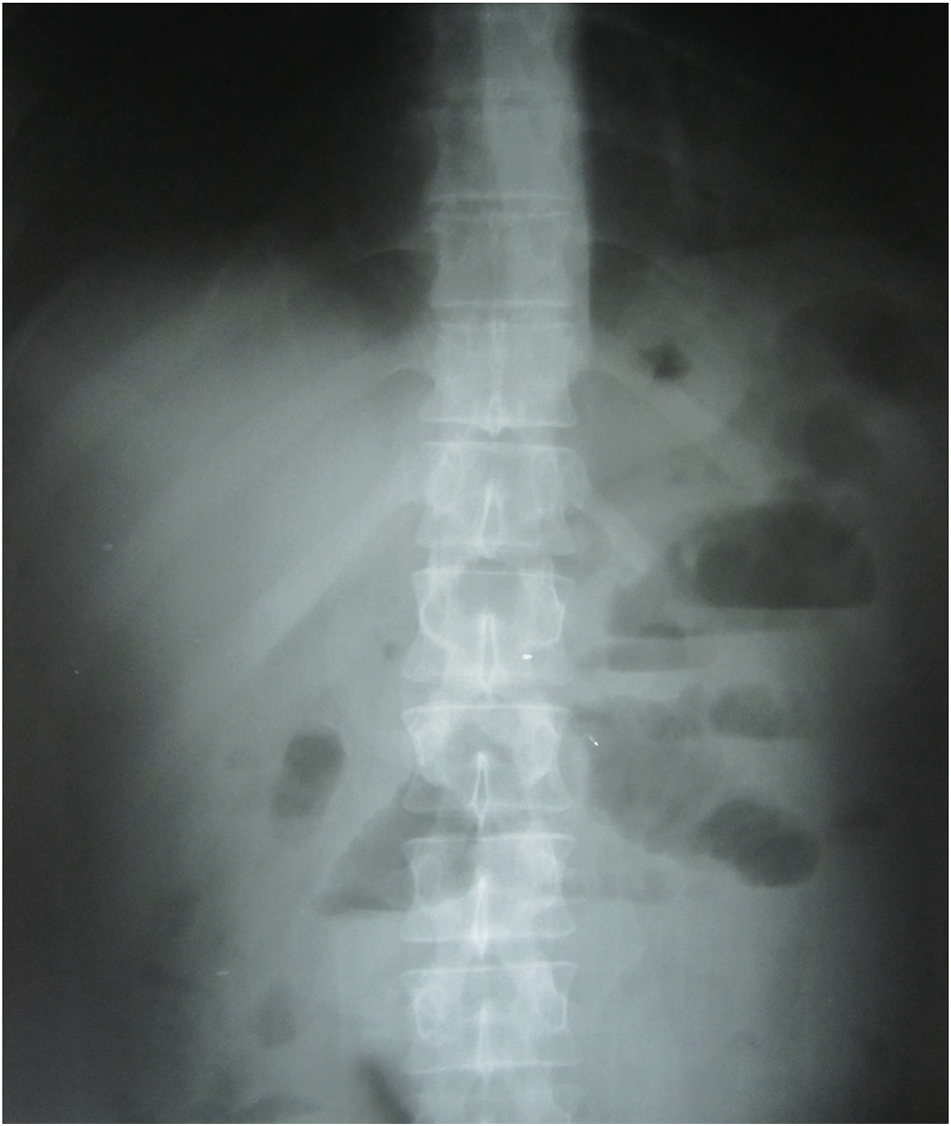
Plain abdominal X-ray in erect position showing multiple air-fluid levels suggesting intestinal obstruction.
